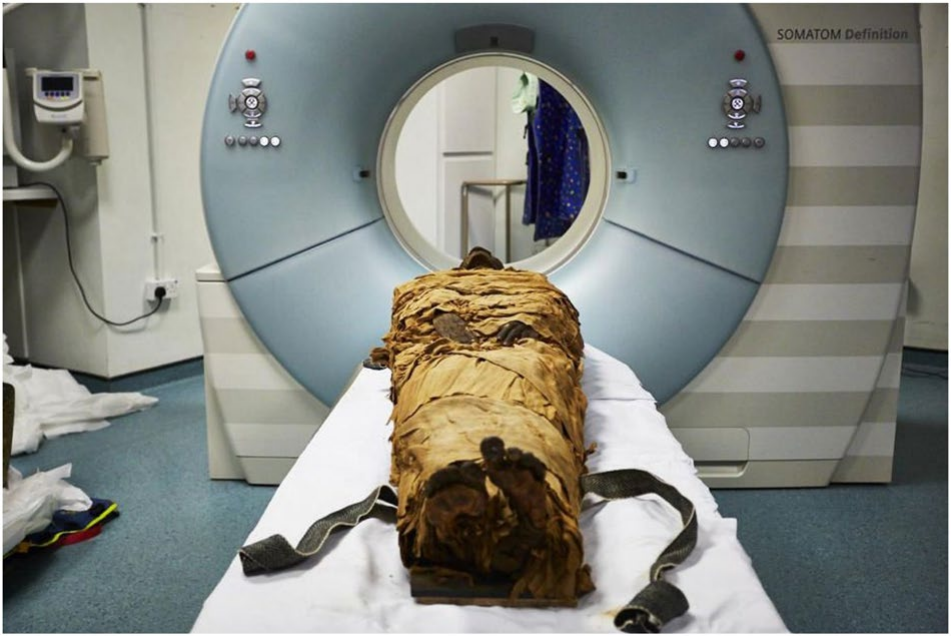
The mummified body of Nesyamun laid on the couch to be CT scanned at Leeds General Infirmary. © Leeds Teaching Hospitals/Leeds Museums and Galleries.
